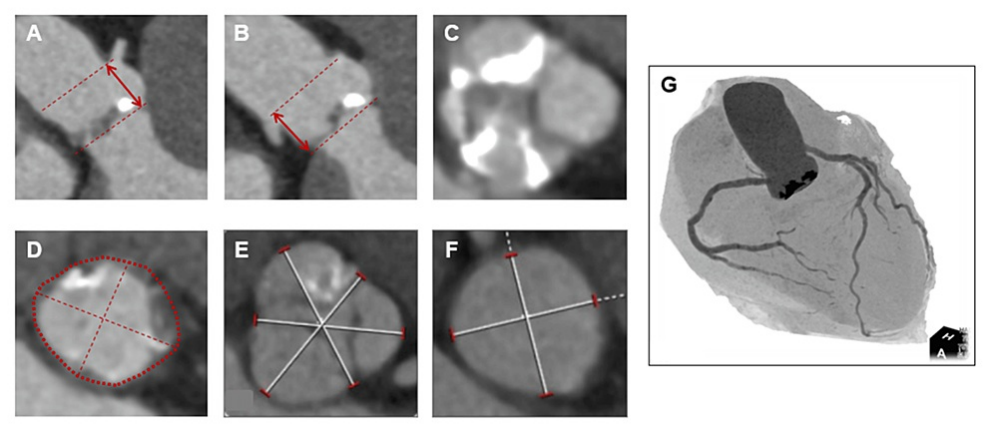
Computed tomography images before TAVI (A) Right coronary artery ostium height: 14.3 mm. (B) Left coronary artery ostium height: 14.8 mm. (C) Severe calcifications on the right and non-coronary cusps. (D) Aortic annulus area: 338 mm^2^, perimeter: 66.1 mm, and diameter: 18.3 mm × 22.1 mm. (E) The sinus of Valsalva: 28.0 mm × 28.5 mm × 28.3 mm. (F) The sino-tubular junction: 24.7 mm×25.3 mm. (G) Angiographic view. TAVI: transcatheter aortic valve implantation
